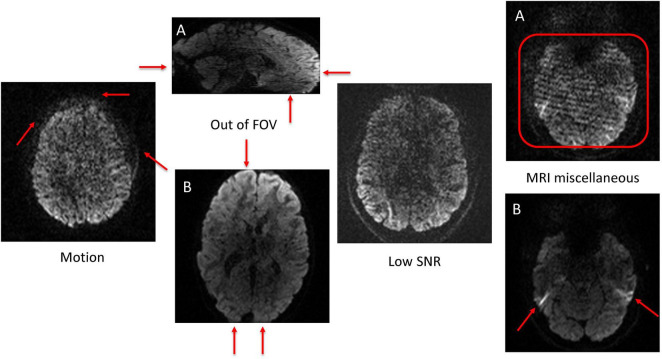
Examples of the four artifact classes. Artifact patterns are demonstrated with red arrows or rectangles. The motion artifact blurring patterns are observable across the border between the brain and the background as depicted by the red arrows. To better observe the out of FOV class, two views of the same volume were depicted [(A): Sagittal and (B): Axial]. The example for the low SNR class, shows a poor signal to noise ratio all over the image. Two different artifact types (form two different volumes) belonging to the MRI miscellaneous artifact class are shown in the right side of the figure [(A): Herringbone style artifacts are evident in the region shown by the red rectangle; (B): Susceptibility artifact are show on both sides of the brain by the red arrows].
